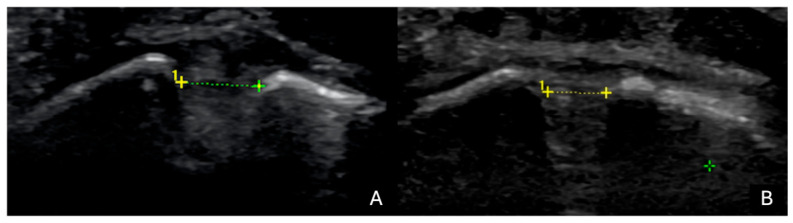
(A) Ultrasound images of the wrist after dynamic maneuvers with support on the head of the third metacarpal. (B) Ultrasound images of the wrist after dynamic maneuvers with the patient clenching their fist.
